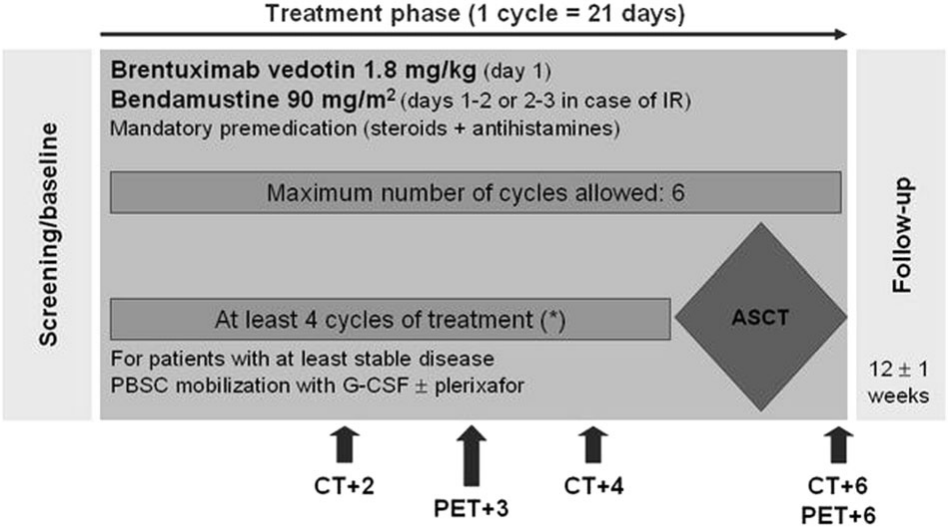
Treatment plan and timing of CT and PET scan assessments. (*) Patients in response were allowed to undergo ASCT after 2 cycles in case of severe toxicity (see text for details). IR, infusion reaction; ASCT, autologous stem cell transplantation; PBSC, peripheral blood stem cells; and G-CSF, granulocyte colony-stimulating factor.
